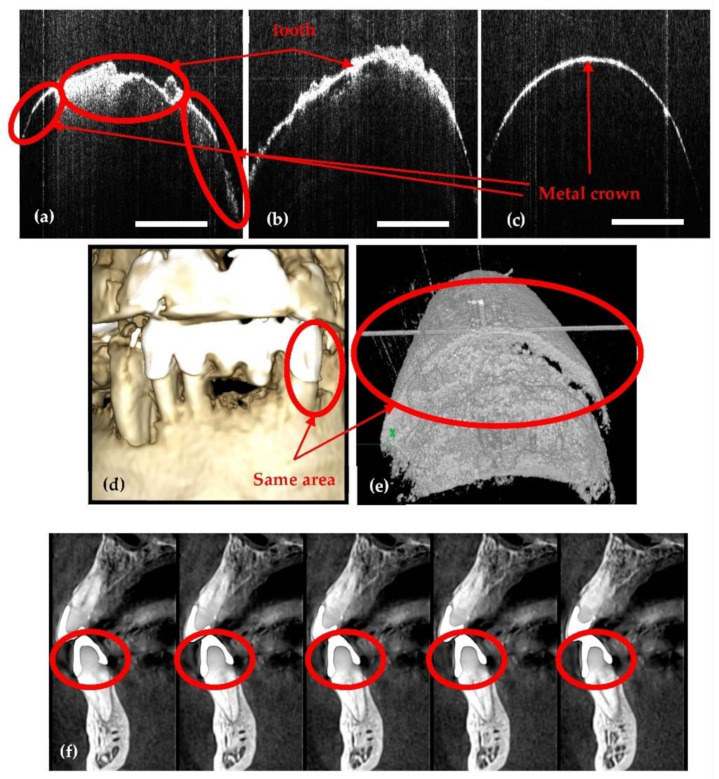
An example where OCT spots dental details near metal crowns. (a–c) OCT B-scans; (d) 3D rendering of CBCT; (e) OCT 3D reconstruction; (f) 3D CBCT sagittal view of the tooth. Scale: 1 mm.
